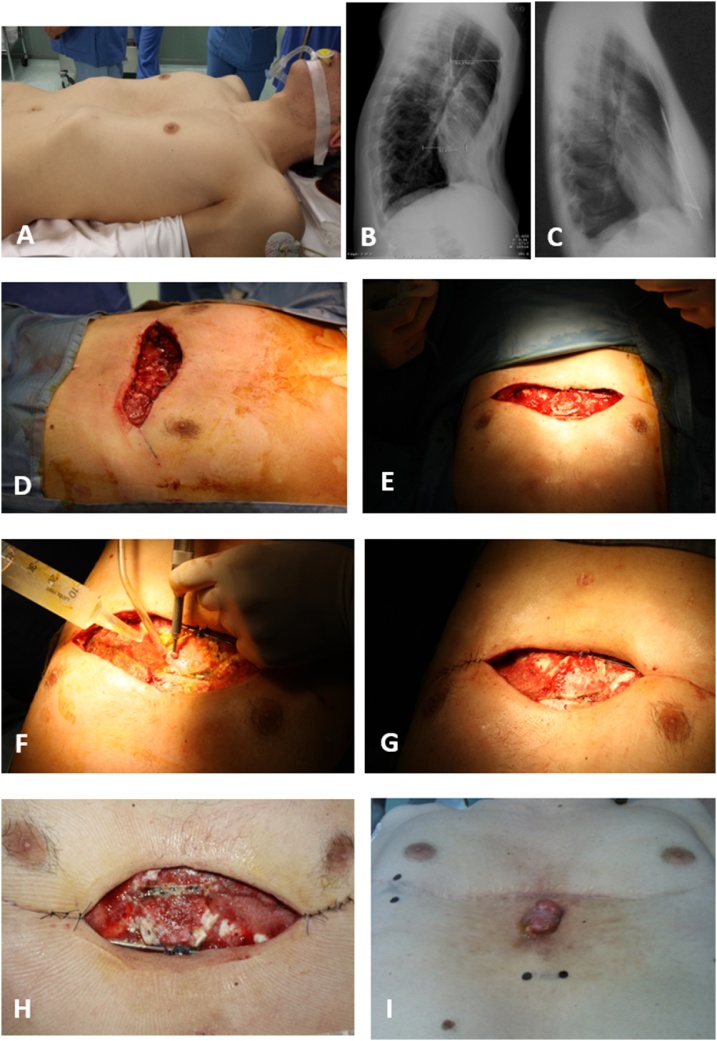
A. Patients before surgery. 1B. Pectus deformity is clearly visible in supine position as well as by chest x-ray. 1C. Chest x-ray after surgery and before bars removal, showing a satisfactory result with no hardware exposure. 1D. The wound before the first-time surgical debridement. 1E. The wound appears red and full of serum. 1F-G. The debridement and medication during the second surgical debridement. 1H. Third time surgical debridement. 1I. The wound appeared less red and without serum. At this time the skin was closed after 10 days of VAC therapy.
